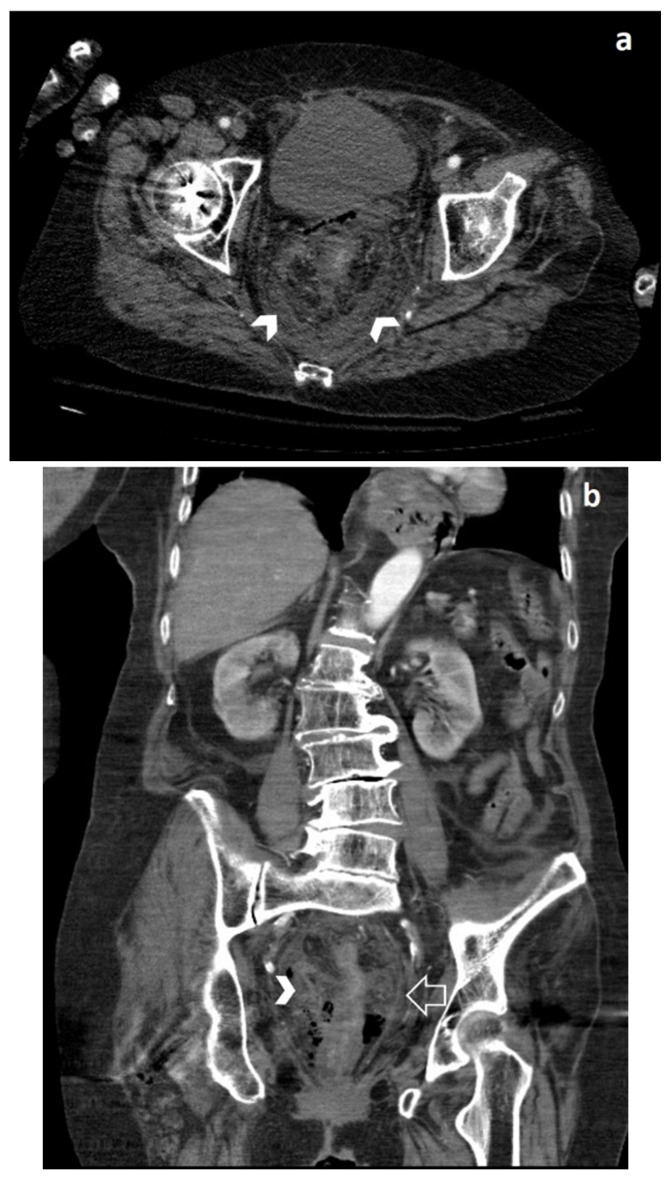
Axial (a) and coronal (b) CT images show perivisceral fat stranding (arrowheads) and thickening of the mesorectal fascia (open arrow in (b)).
